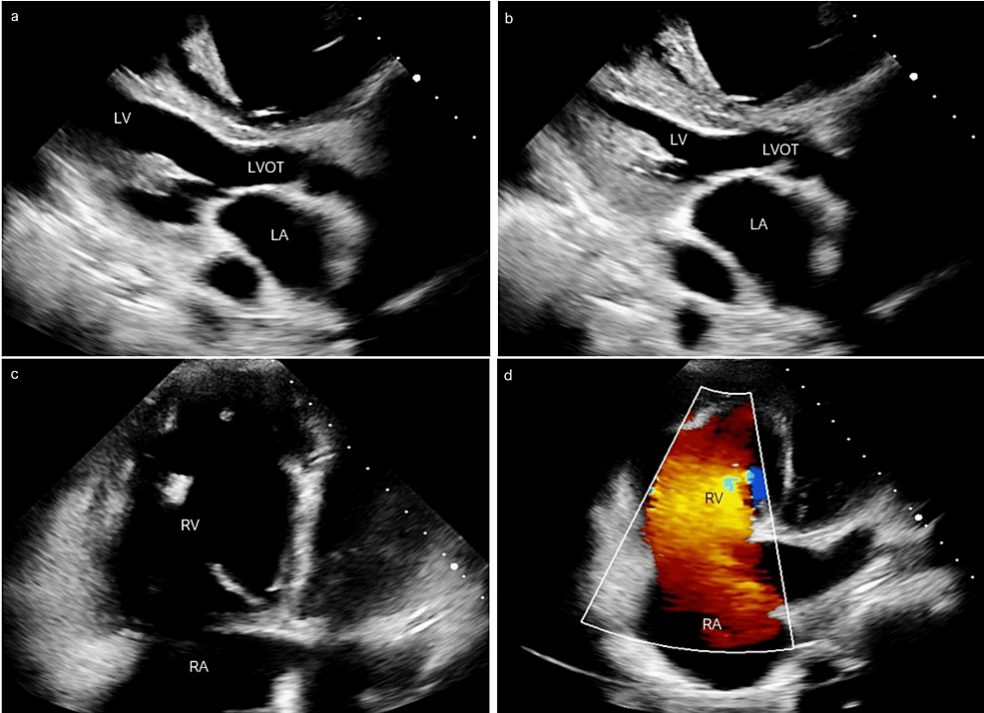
Transthoracic echocardiogram, parasternal long-axis view. (a, b) Show severely underfilled LV at diastole with normal size and EF, respectively. (c, d) Show severely dilated RV with mildly reduced function and high flow through the right side of the heart, respectively. LV, left ventricle; EF, ejection fraction; RV, right ventricle; LA, left atrium; RA, right atrium; LVOT, left ventricular outflow tract.
